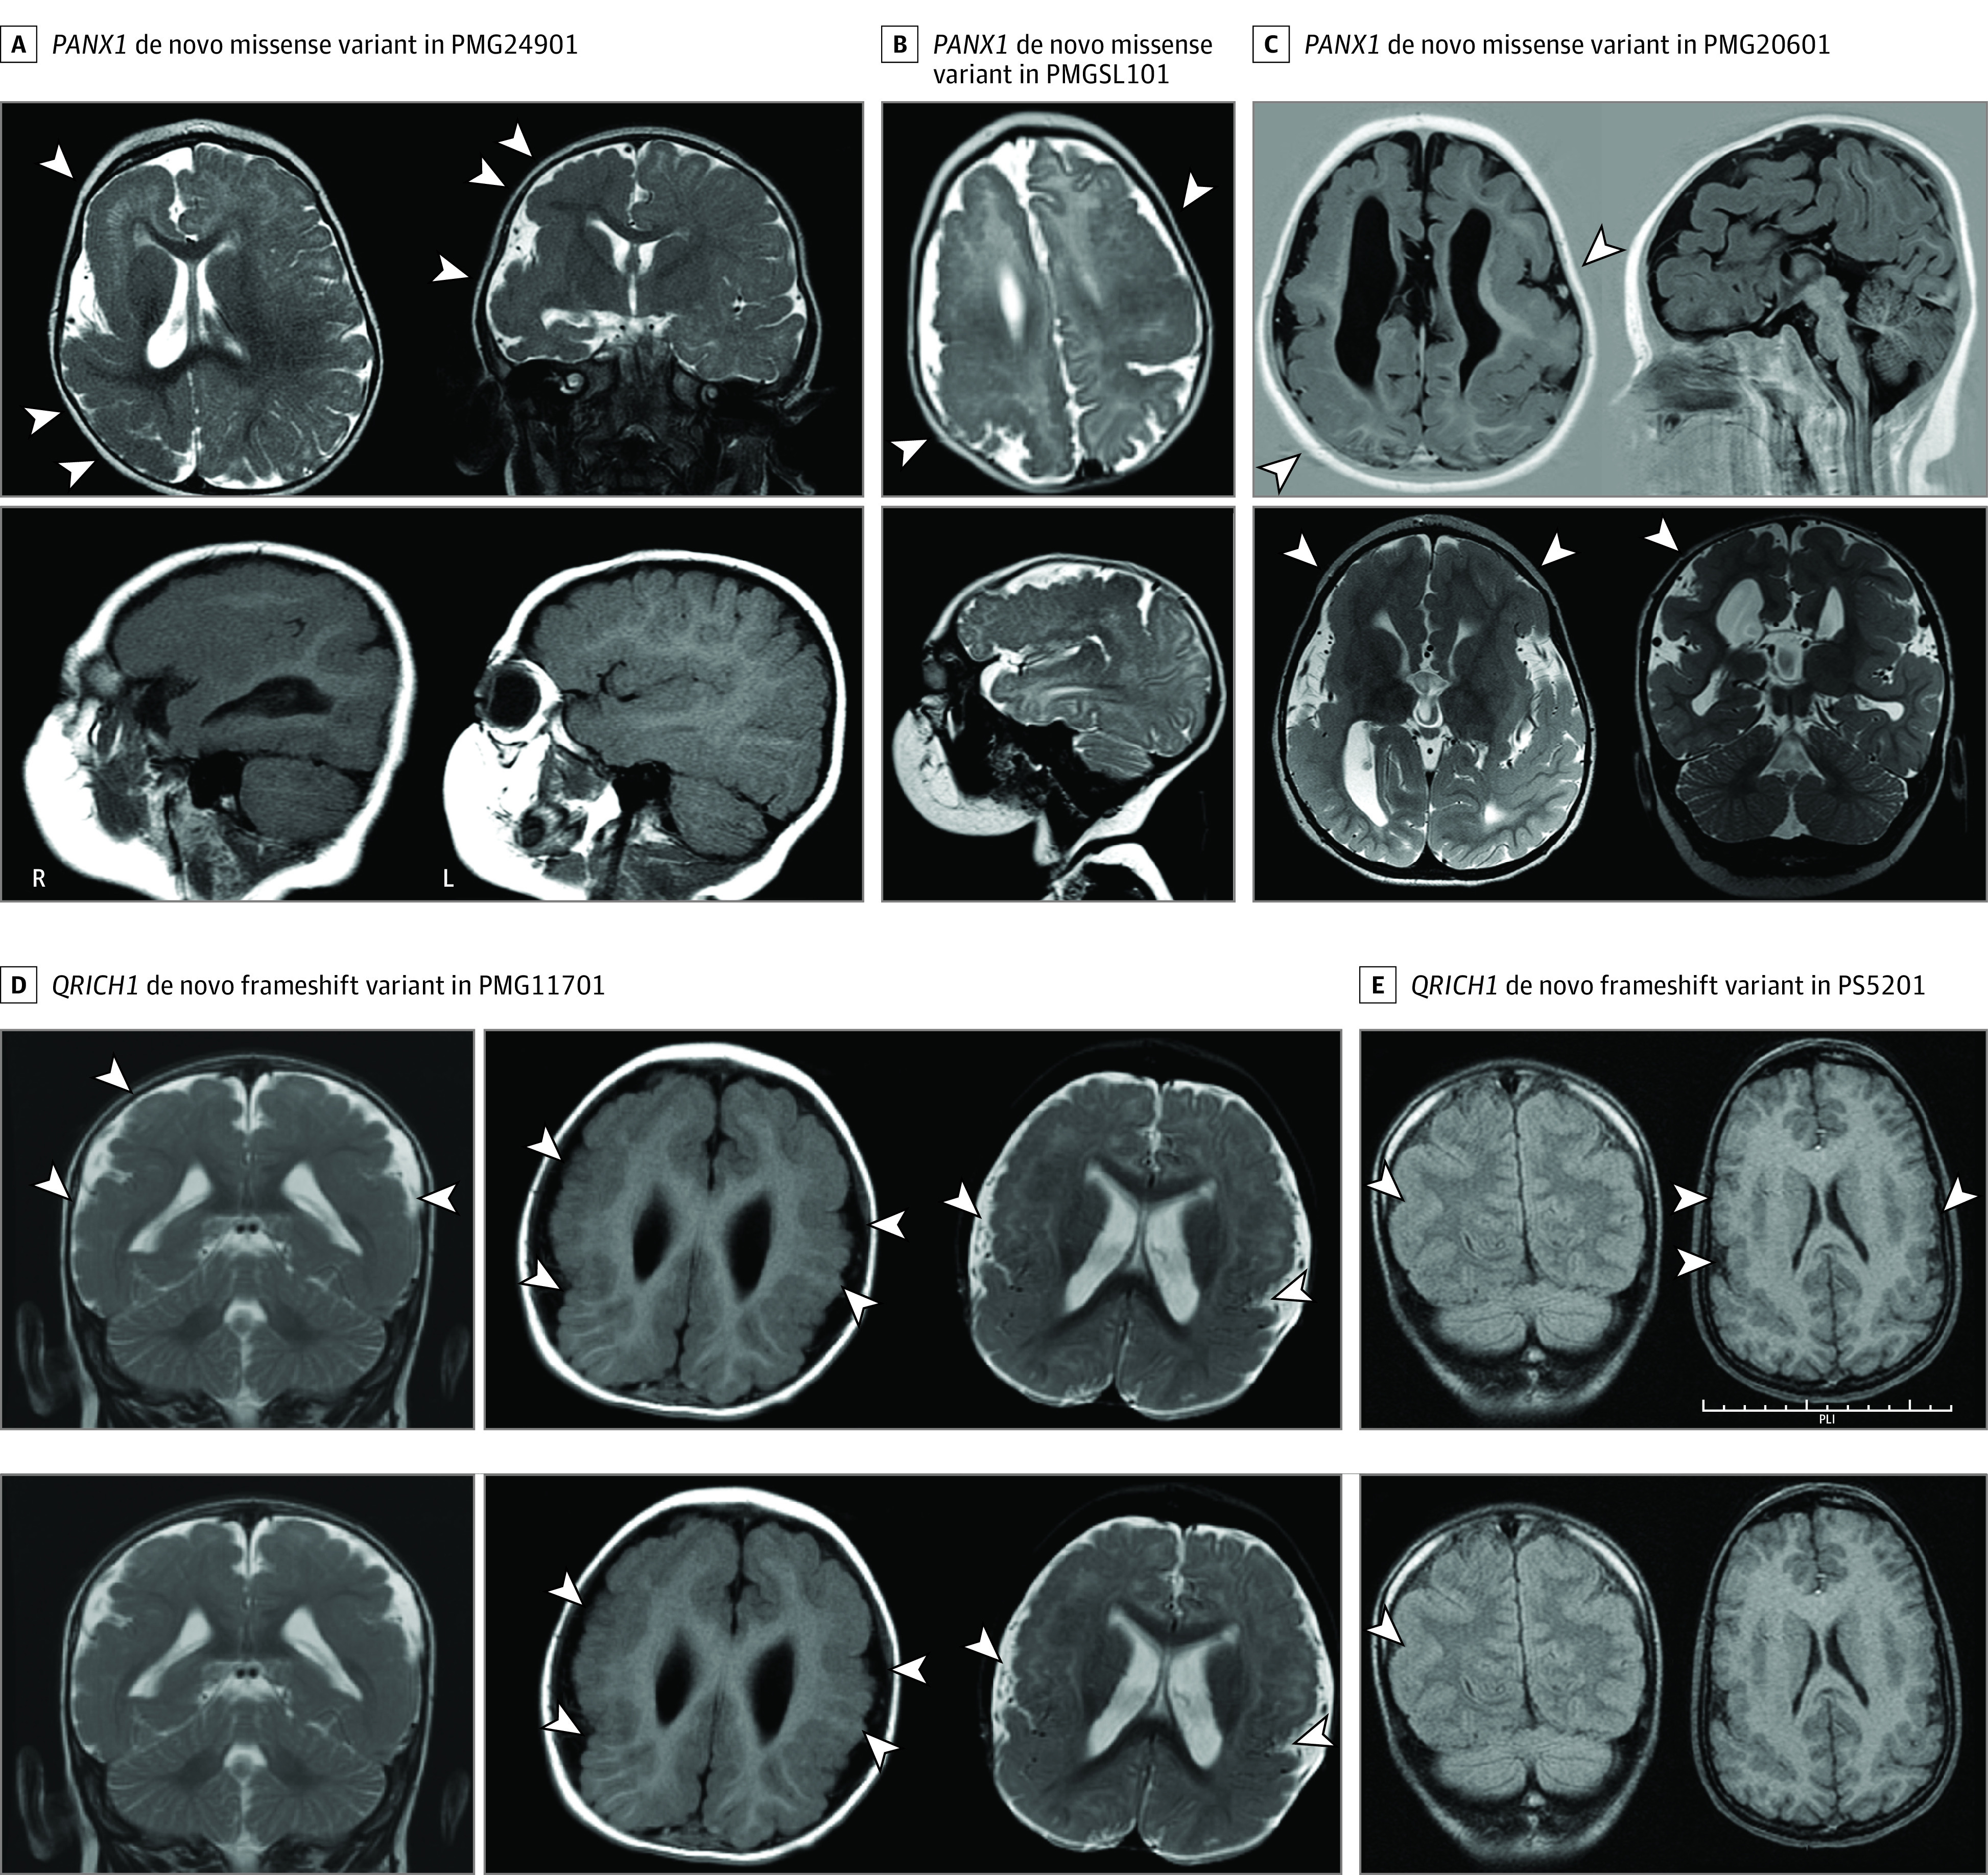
Magnetic Resonance Images From Affected Individuals With *PANX1* and *QRICH1* Variants A, PMG24901 has a de novo missense variant in *PANX1* (p.Asn338Thr) and unilateral right severe polymicrogyria and agyria with an unremarkable left hemisphere. B, PMGSL101 has a de novo missense variant in *PANX1* (p.Met37Arg) and has diffuse bilateral polymicrogyria with closed lip schizencephaly. C, PMG20601 has a de novo missense variant in *PANX1* (p.Asp14His) as well as diffuse bilateral polymicrogyria, worse on the right than left, and poor myelination. D, PMG11701 has a de novo frameshift variant in *QRICH1*, p.Phe384GlnfsTer5. E, PS5201 has a de novo frameshift variant in *QRICH1*, p.Val102PhefsTer144. Example regions of polymicrogyria are marked with white arrowheads.
